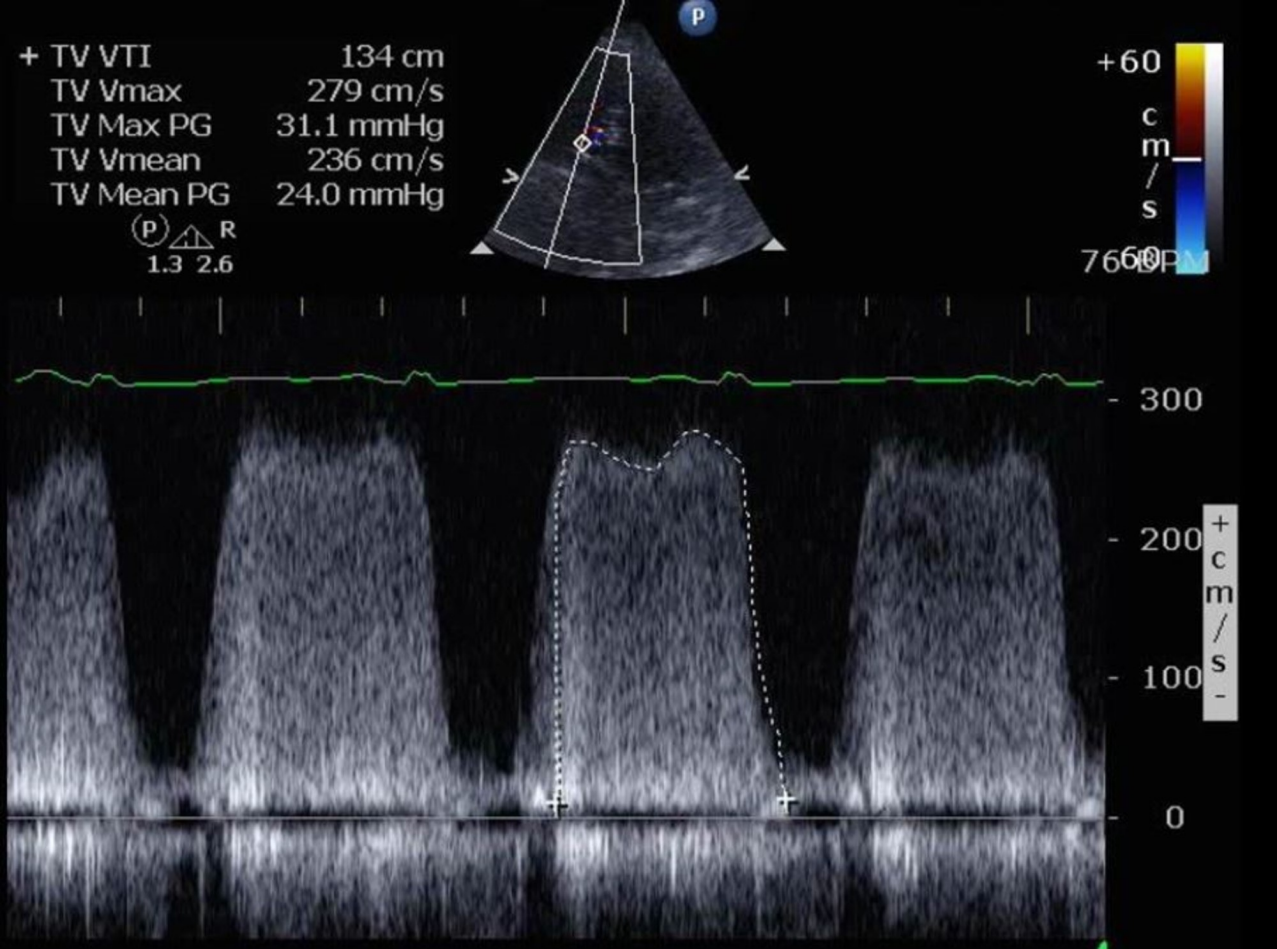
Continuous wave Doppler across the tricuspid valve; mean gradient is 24 mmHg
